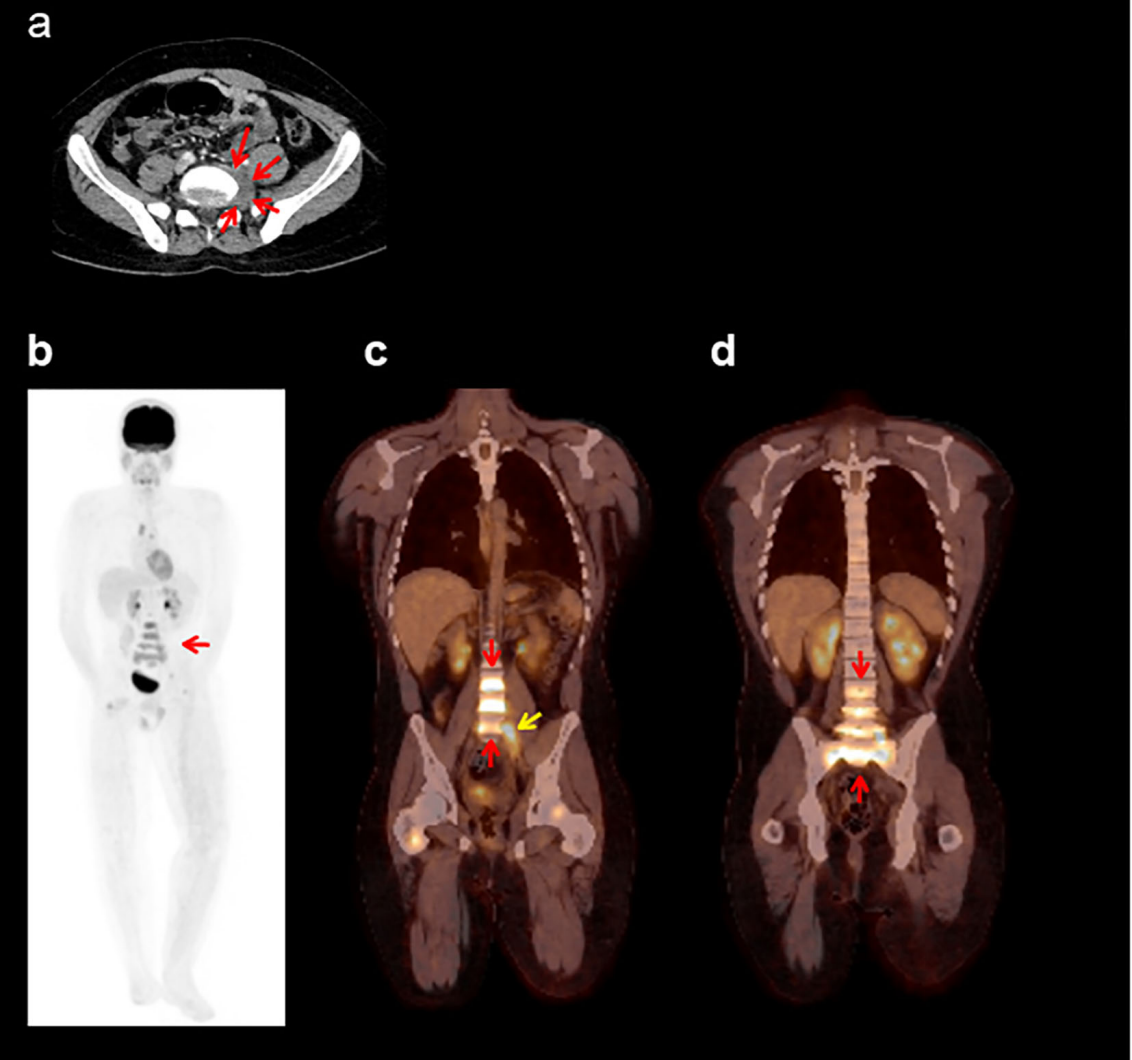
Tumor at progressive disease. (a) CT scan indicating progressive disease in the left paravertebral soft tissue mass (red arrows). (b–d) Images correspond to the PET-CT study at progressive disease—MIP image (b) and coronal fusion images (c, d)—which show a diffuse increase in metabolism in the lumbosacral vertebrae, encompassing the entirety of the vertebral bodies (red arrows), as well as hypermetabolism associated with the soft tissue mass in the left paravertebral region at L5 (yellow arrow). CT, computed tomography; MIP, maximum intensity projection; PET, positron emission tomography.
